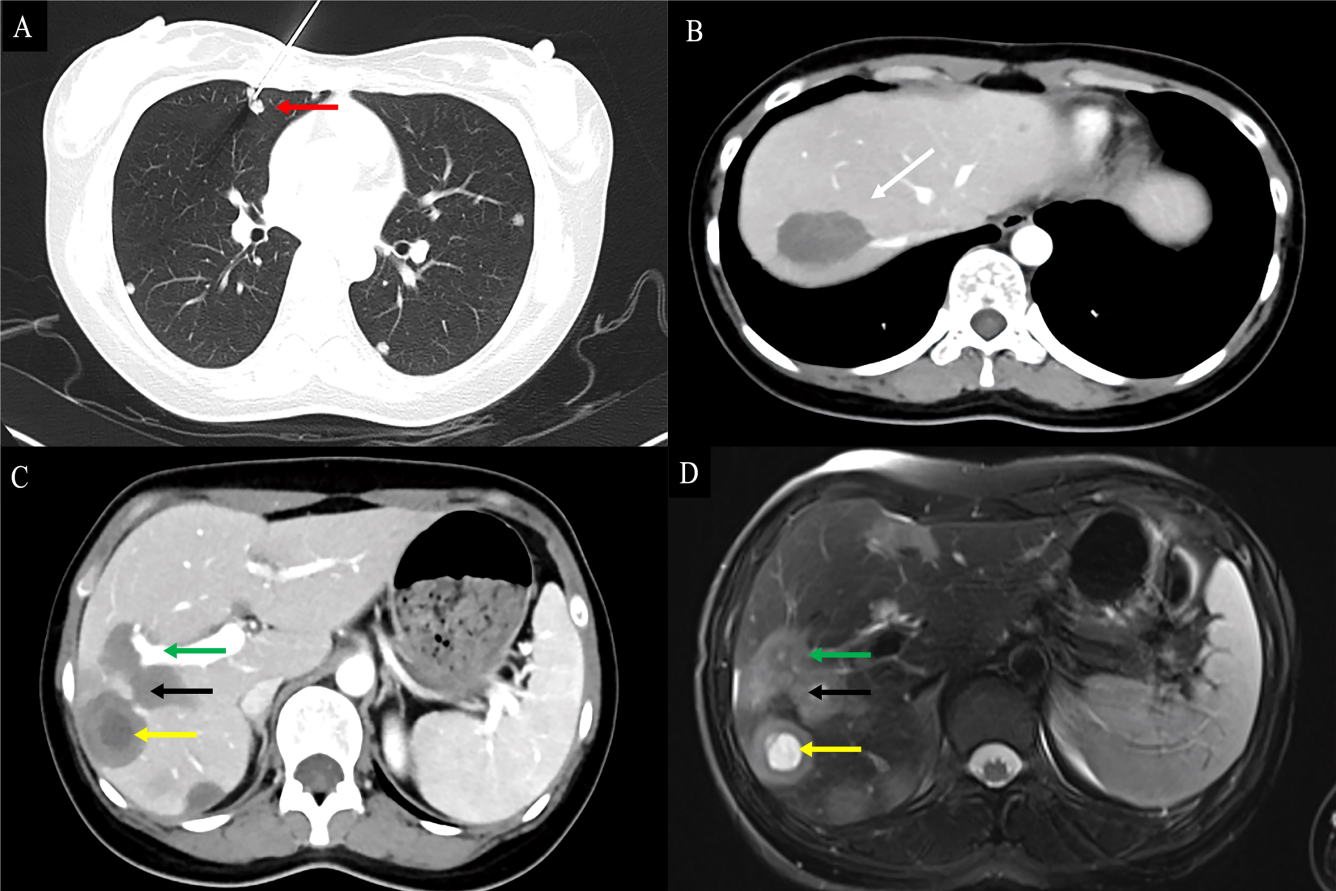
Findings of HEHE in contrast-enhanced CT and contrast-enhanced MRI. A 26-year-old female patient with HEHE was undergoing CT-guided FNA (A). The image of abdominal contrast-enhanced CT demonstrated a peripherally located characteristic “lollipop sign” (white arrow) (B) and a “target sign” (yellow arrow) associated with invasion of the RPV (green arrow) and coalescent lesions (black arrow) (C). The corresponding T2WI demonstrated the characteristic “target sign” (yellow arrow) with coalescent lesions (black arrow) (D). HEHE, hepatic epithelioid hemangioendothelioma; CT, computed tomography; MRI, magnetic resonance imaging; FNA, fine needle aspiration; RPV, right branch portal vein; T2WI, T2-weighted image.
